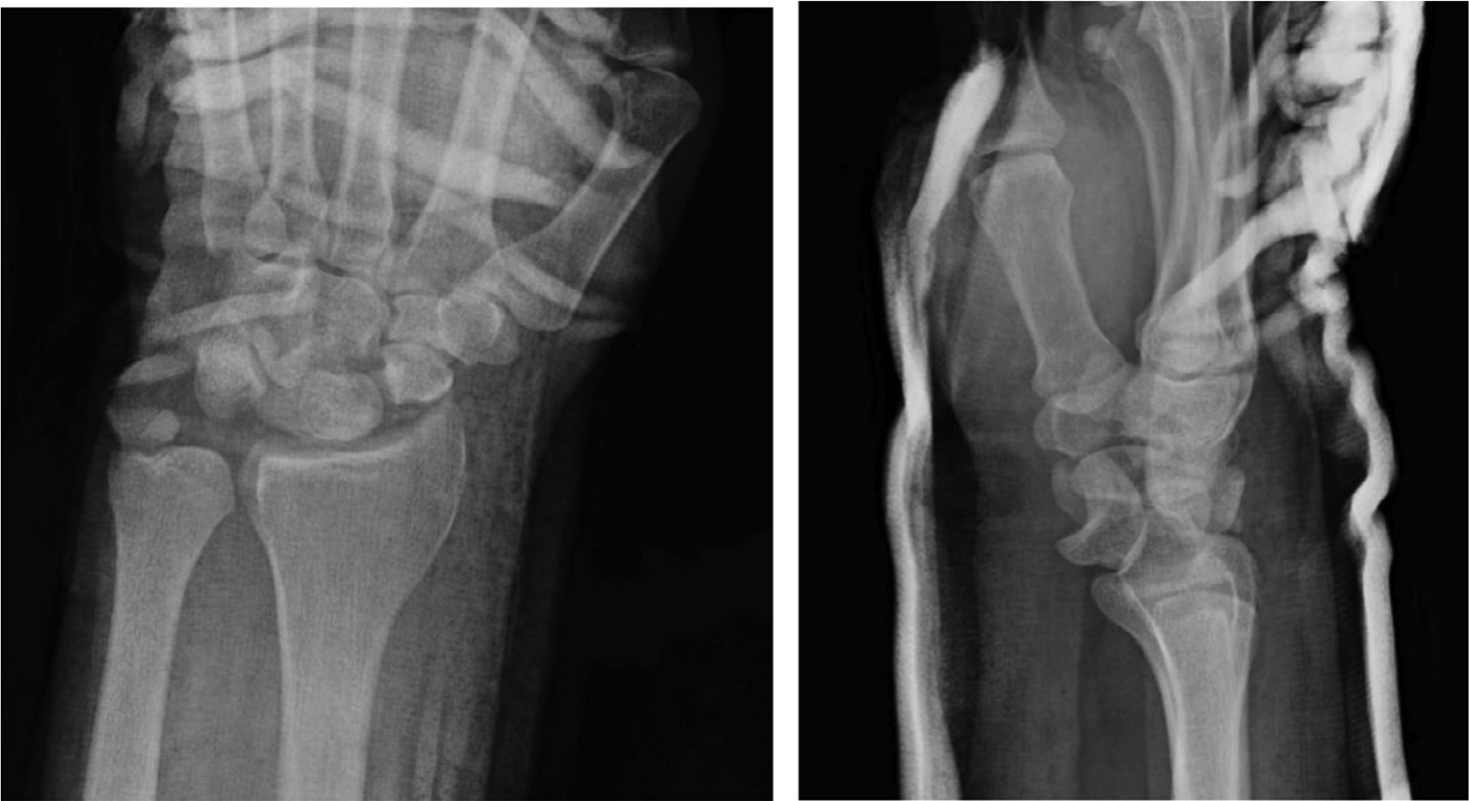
Patient 2: Initial radiographs of the left wrist demonstrate trans-ulnar styloid trans-capitate trans-scaphoid lunate dislocation. Overlying splint material is present.
